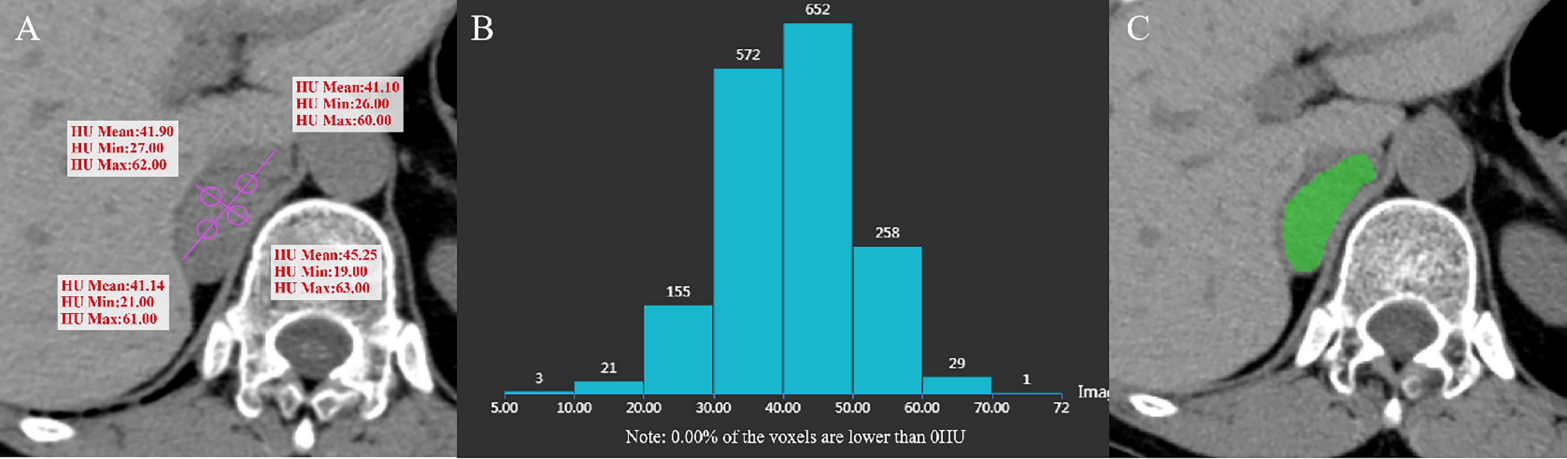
Pheochromocytoma of the right adrenal gland in a 64-year-old woman. AV_mean_ = 41.345 HU, AV_min_ = 23.25 HU (A), and CTH negative pixel = 0 (B). CT value in the green area is > 10 HU (C).
